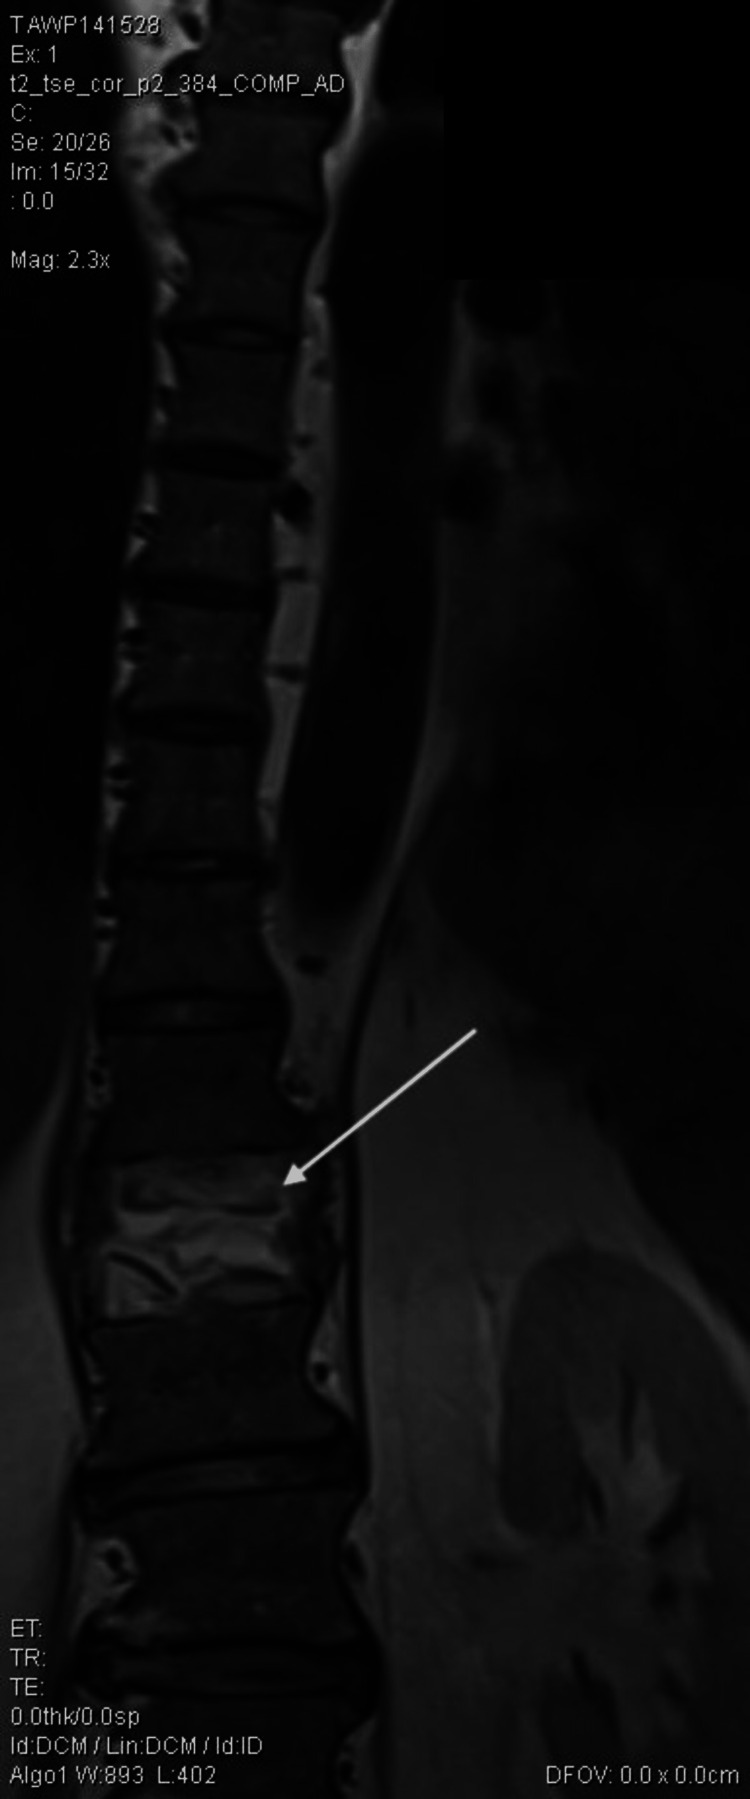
Magnetic resonance imaging of the spine in coronal view. The white arrow indicates compression fracture.
